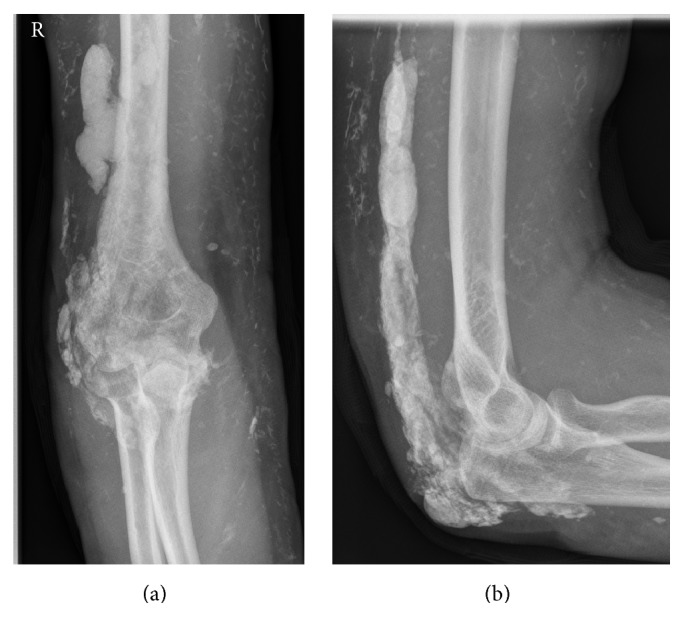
Standard elbow radiographs of a 24-year-old woman with calcinosis universalis in juvenile Dermatomyositis. (a) Anterior-posterior radiograph, (b) lateral radiograph.
